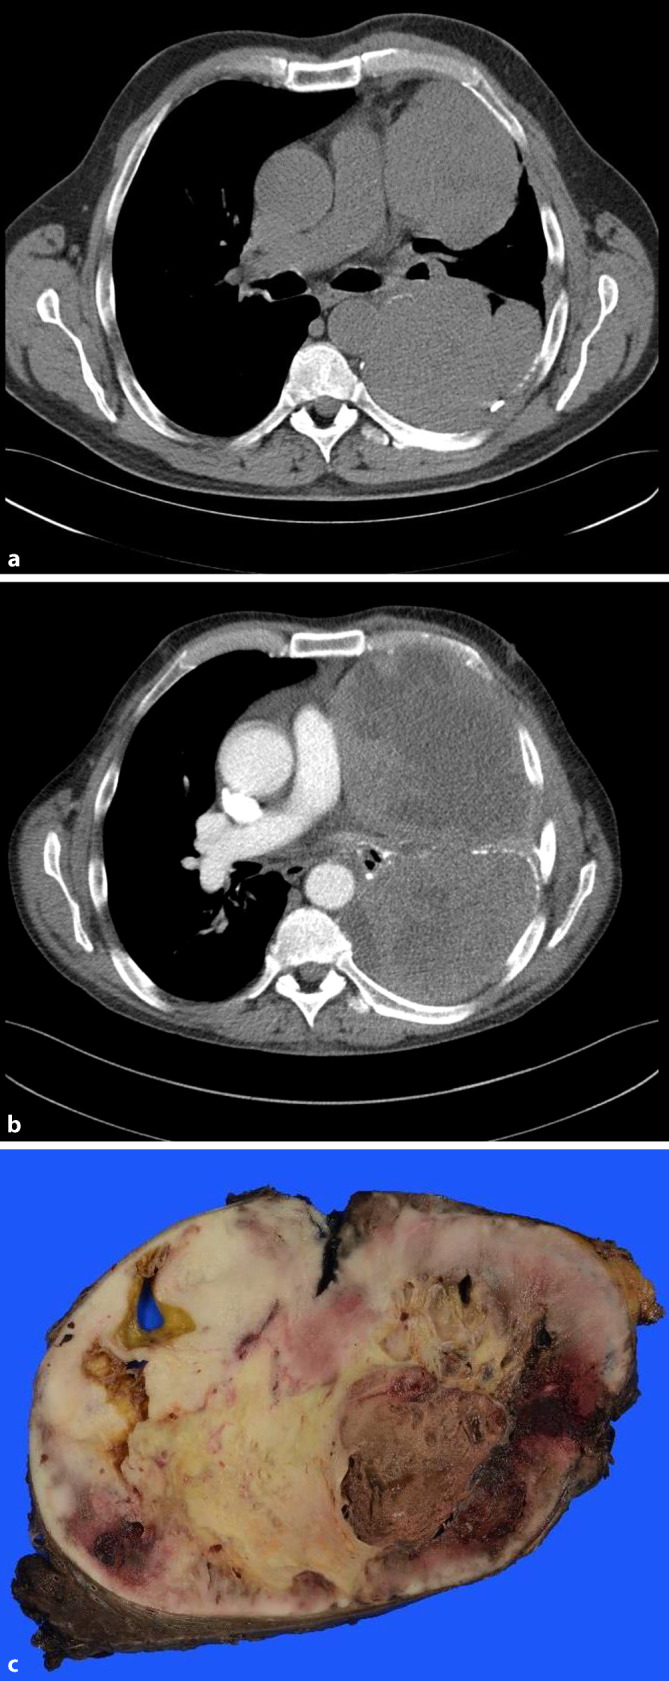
Case 1: Computed tomography scan of the thoracic relapsed tumor and macroscopy of the resected tumor. a Two new tumor nodules in the left thorax in July 2018. b Tumor progression in November 2018. c Resected tumor after 5‑month mTOR inhibitor treatment
